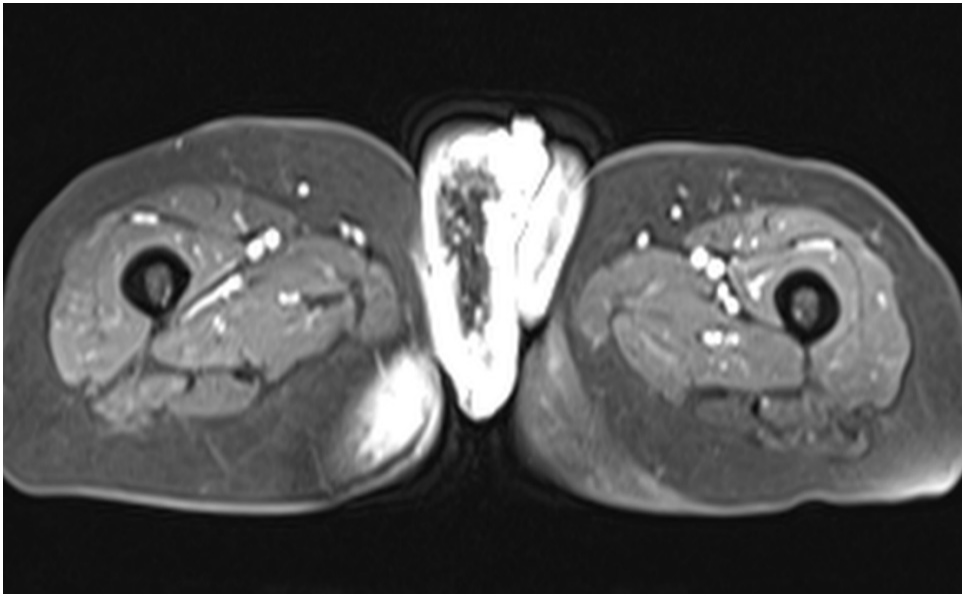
Pelvic MRI showed significant thickening of the vulvar mass and multiple enlarged surrounding lymph nodes.
